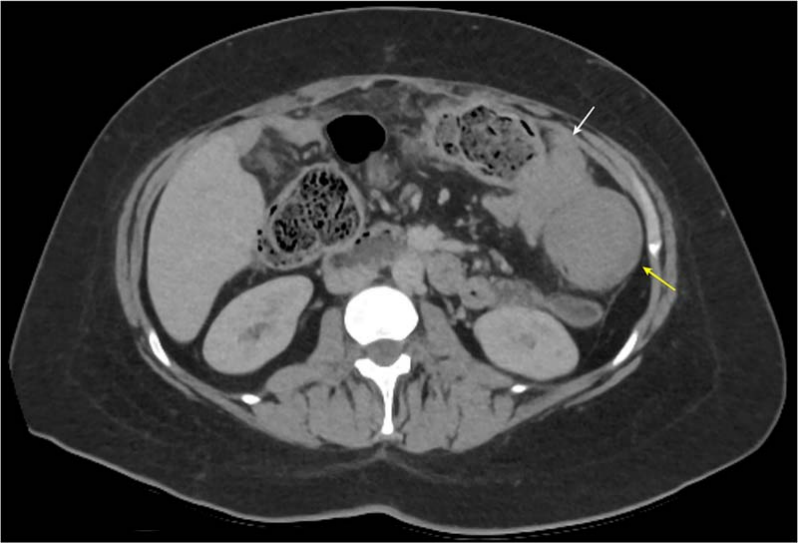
Abdominal CT axial slice demonstrating colo-colonic intussusception at the splenic flexure and a colonic soft tissue mass just distal.
